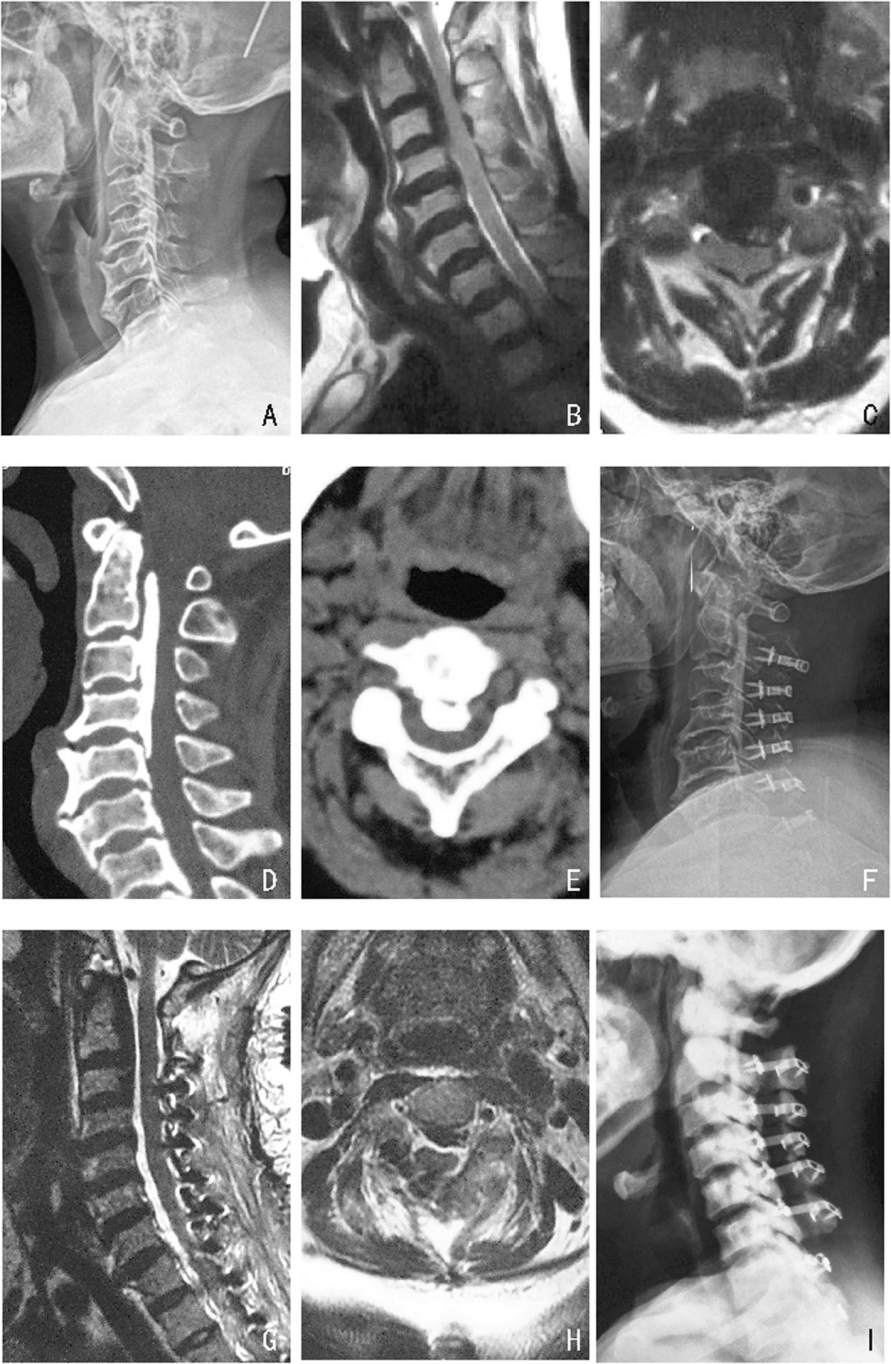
a, f, i A comparison of the changes in cervical alignment observed between measurements among preoperative, postoperative and at 1 year after the operation in radiographs pf a 54-year-old female patient who underwent EODL Extended to C2. b-e Preoperative MRI and CT. g-h Postoperative MRI
